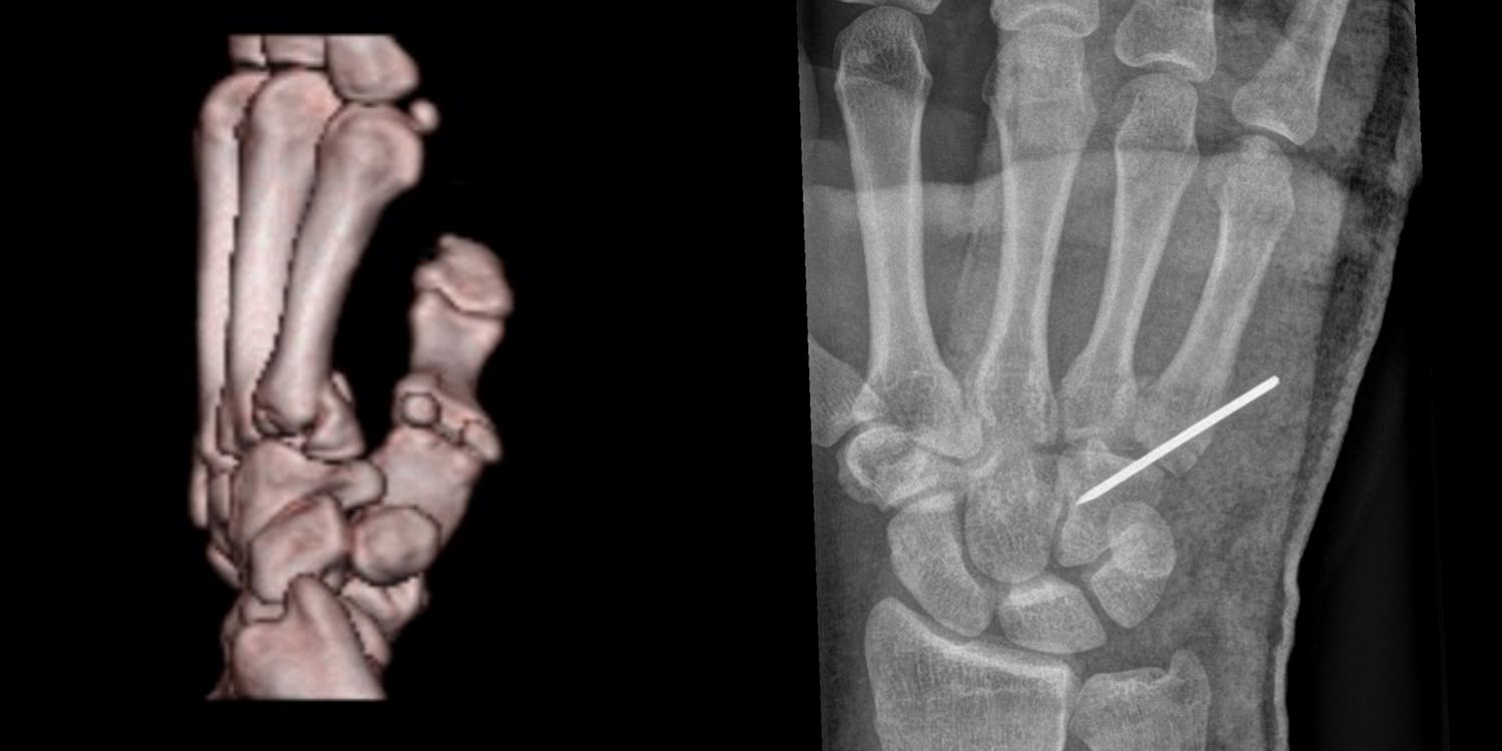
a Preoperative CT-scan revealing a metacarpal five fracture. b Postoperative p/a radiograph following pin fixation
